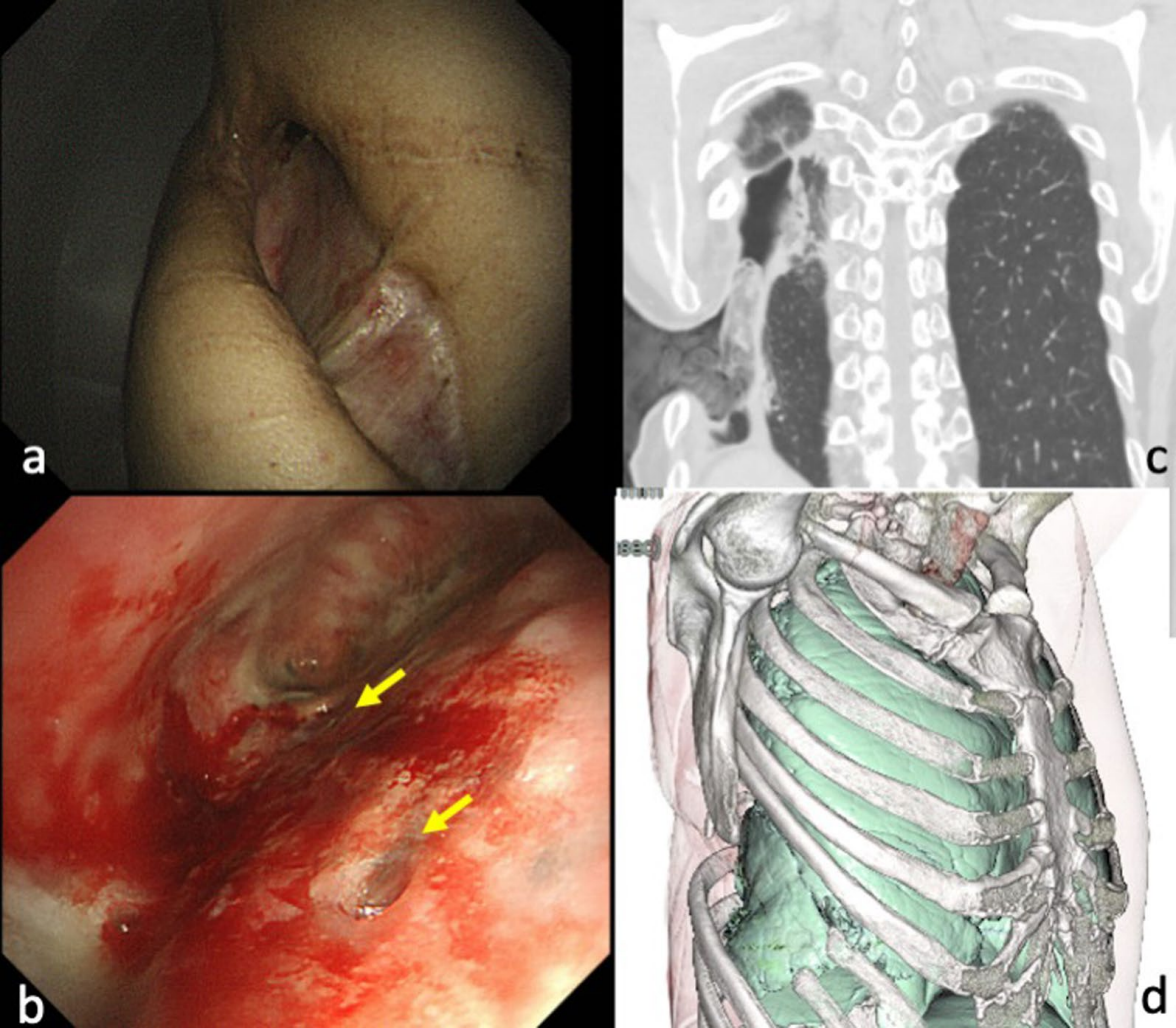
Aspect of open window on the right side of the thorax because of pyothorax. a At least two fistulae were observed (arrows) (b). CT revealed resection of 7th and 8th ribs for open window thoracotomy and cranial extension of the pyothorax cavity (c, d)
